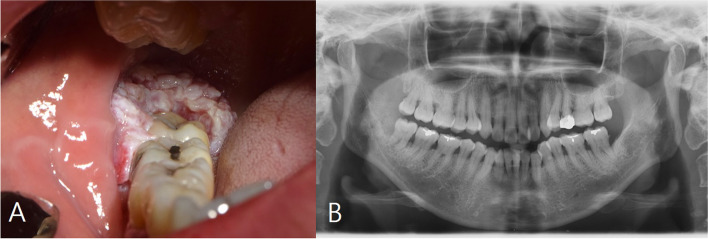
Intraoral assessment of patients in initial visit. A Preoperative intraoral examination presenting whitish exophytic lump on right retromolar area. B Preoperative panoramic radiograph view
